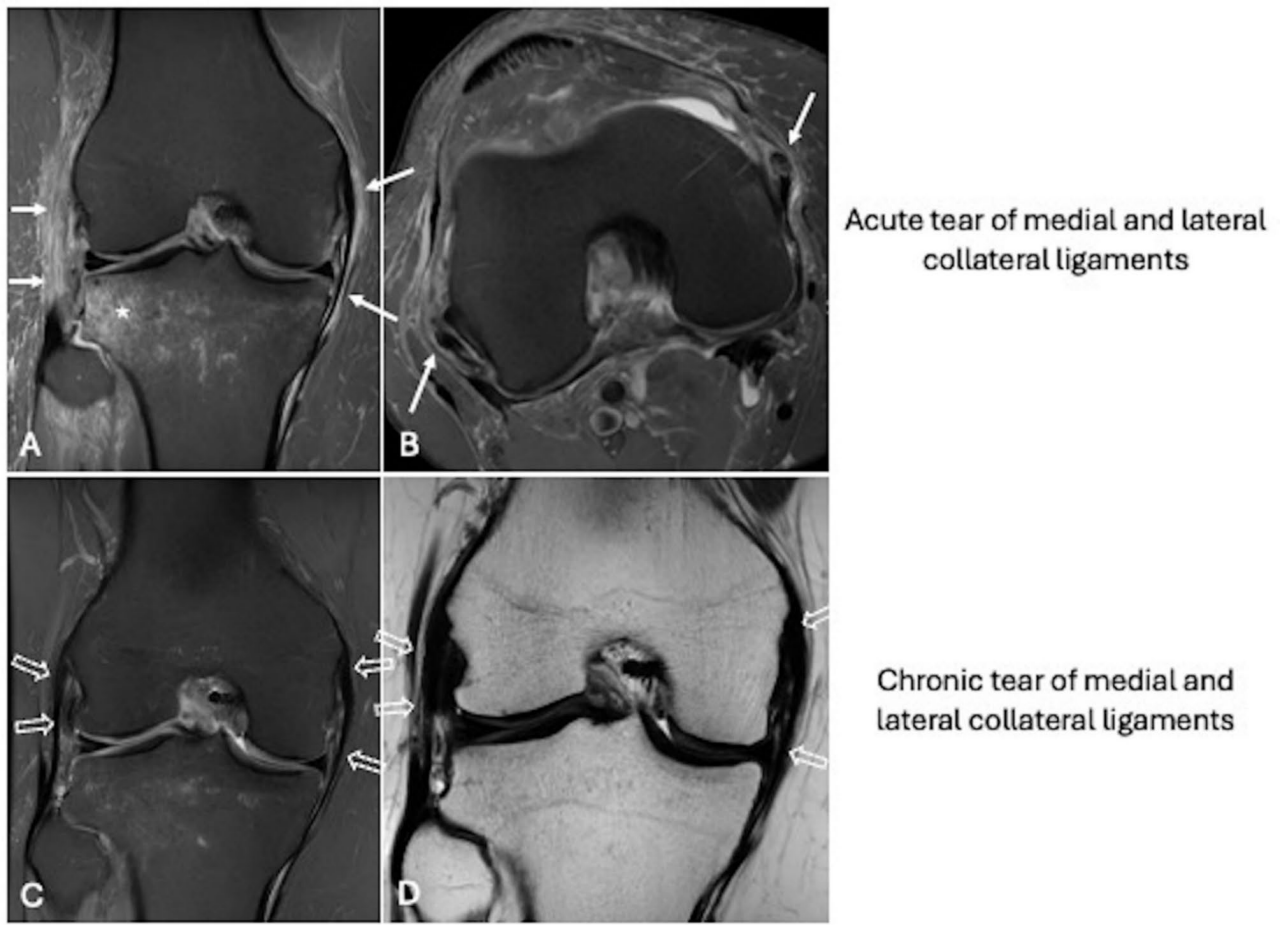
Acute and chronic tear of collateral ligaments. Knee MRI performed 12 days after trauma shows acute tear of medial and lateral collateral ligaments presenting with diffuse peri-ligamentous edema (white arrows) on coronal (A) and axial (B) fat-suppressed proton density weighted images, peri-capsular edema, and lateral tibia bone edema (A; asterisk). Knee MRI performed 5 months after trauma shows chronic tear of medial and lateral collateral ligaments presenting with hypointense thickening of both ligaments (C, D; void arrows) with neither peri-ligamentous edema, nor peri-capsular edema, nor bone edema
